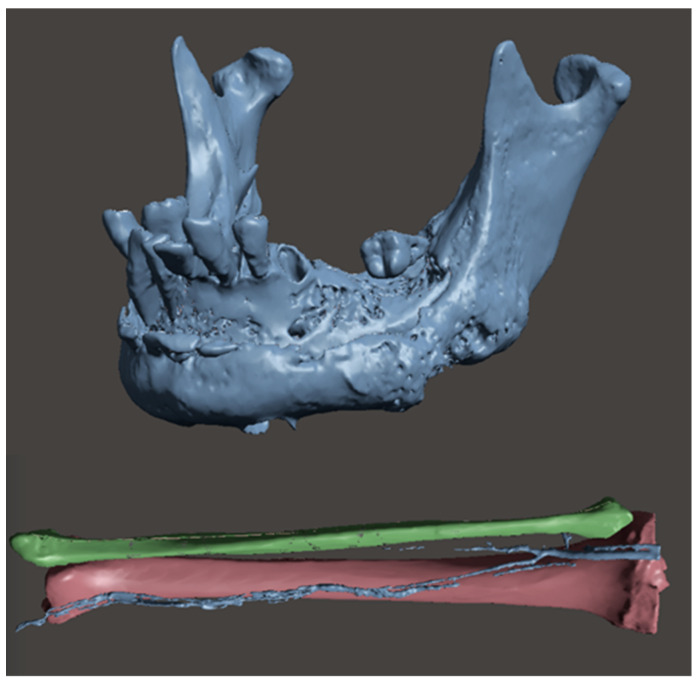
CT data acquisition of the mandible ad fibula.
